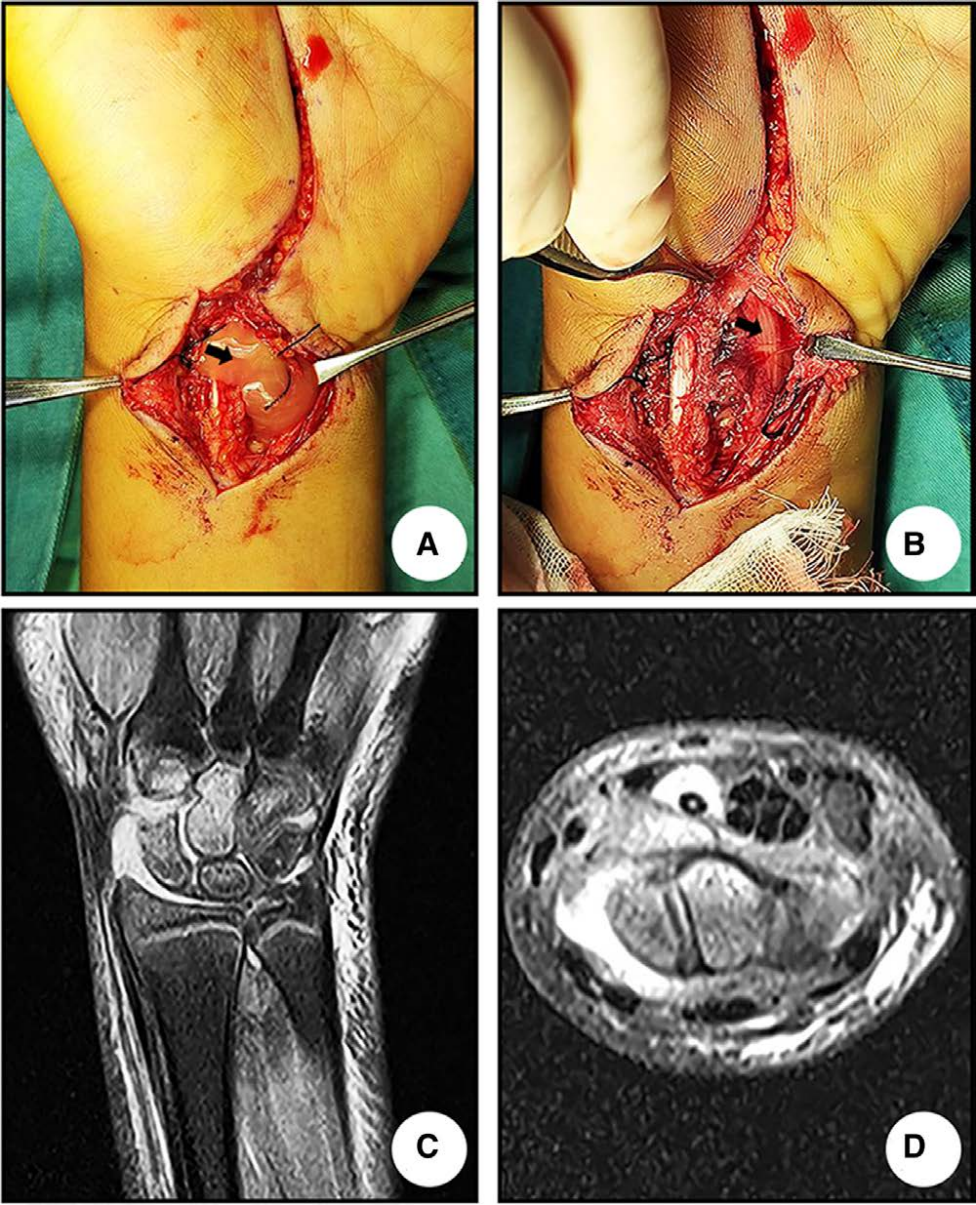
Surgical photo documentation and case imaging. (A) A large amount of yellow-brown pus overflow was seen between the radial carpal flexor and palmaris longus muscles. (B) Pale edematous median nerve. (C) Preoperative MRI coronal view of the left wrist showed bone marrow edema of the carpal bone with a large amount of high signal fluid around it. (D) Preoperative MRI axial view of the left wrist showed marked edema of the soft tissues in the carpal joint cavity, and effusion in the tendon sheath of the bunion spreader muscle and the carpal tunnel.
